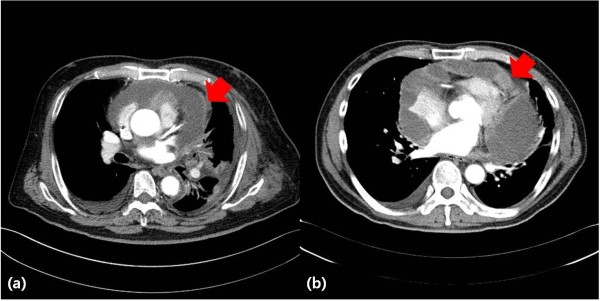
Computed tomography finding suspicious of pericardial metastasis. (a) Pericardial wall thickening (arrow) and (b) pericardial nodularity (arrow).
